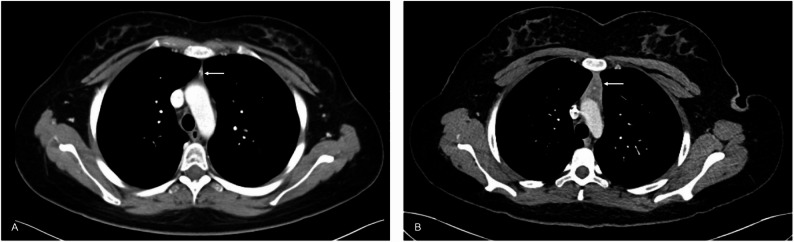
Thymus enlargement after CAR T-cell therapy in a 16-year-old female patient with B-ALL. A) Baseline axial contrast-enhanced chest CT shows a small amount of thymic tissue in the anterior mediastinum (arrow). B) Axial CT image at day 52 after CAR T-cell therapy demonstrates benign thymic enlargement with smooth contours and mild, homogenous contrast enhancement (arrow). The patient demonstrated a robust tumor response with relapse-free survival for four years
